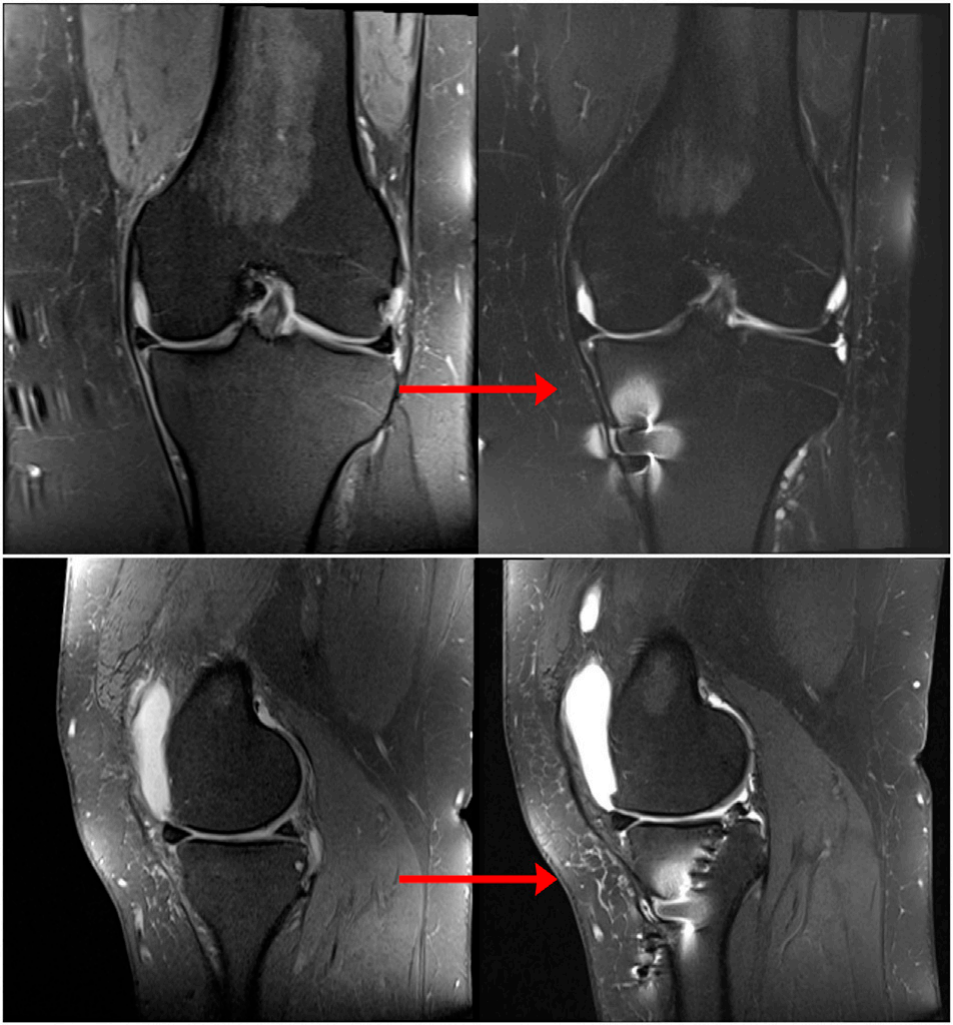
Coronal (A) and sagittal (B) MR images of nonanatomic root repair patient #6 showing progression of osteoarthritis in the medial compartment of the knee over 12 months secondary to nonanatomic medial meniscus root repair (left knee). M = medial; L = lateral; A = anterior; P = Posterior.
